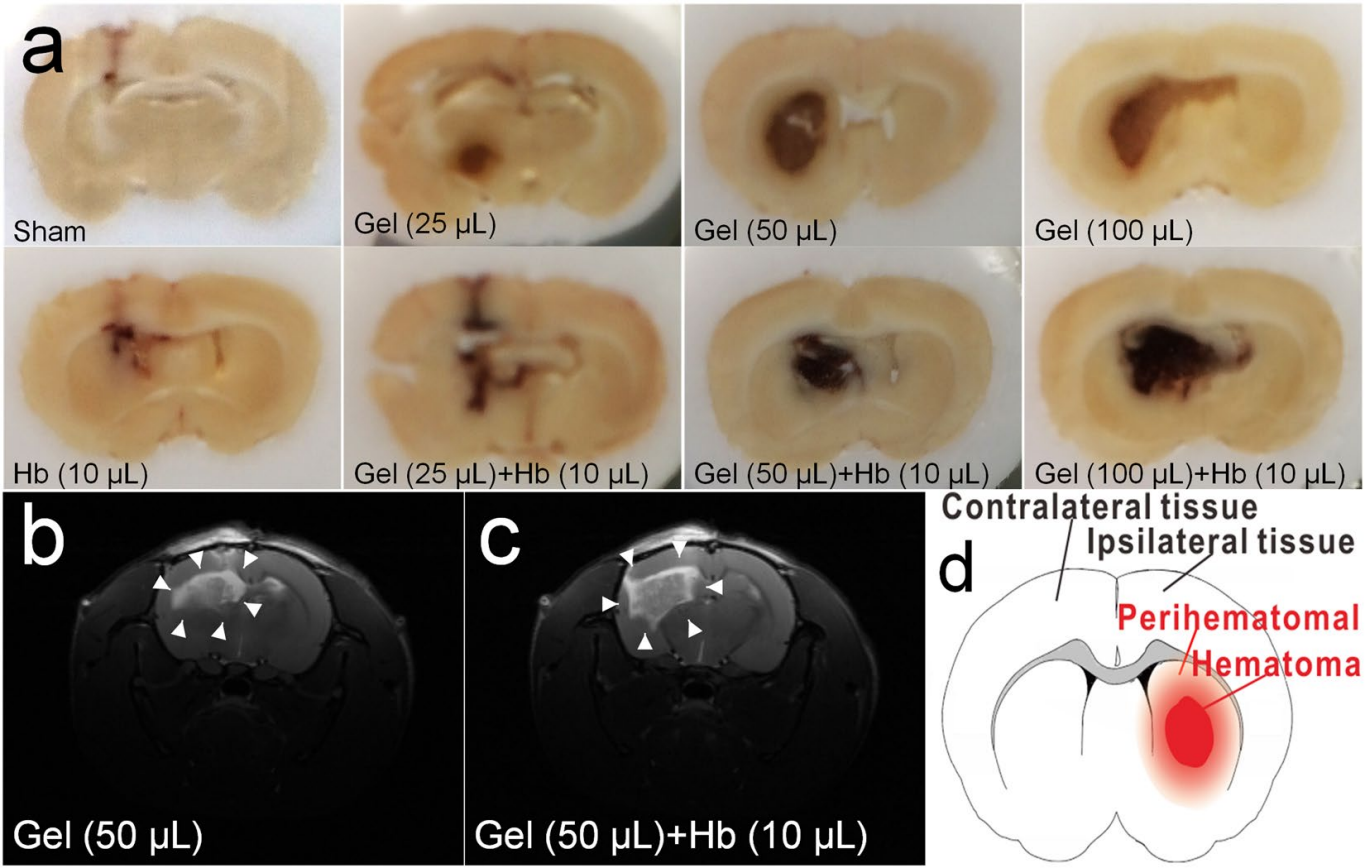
The images of coronal plane and MRI following ICH model after 24 h. (a) The images of coronal plane showed the insults from the agarose gel and hemoglobin on neural tissues. (b,c) Lesions were observed on MRI imaging (white arrows). (d) The hematoma and perihematomal tissues following ICH.
